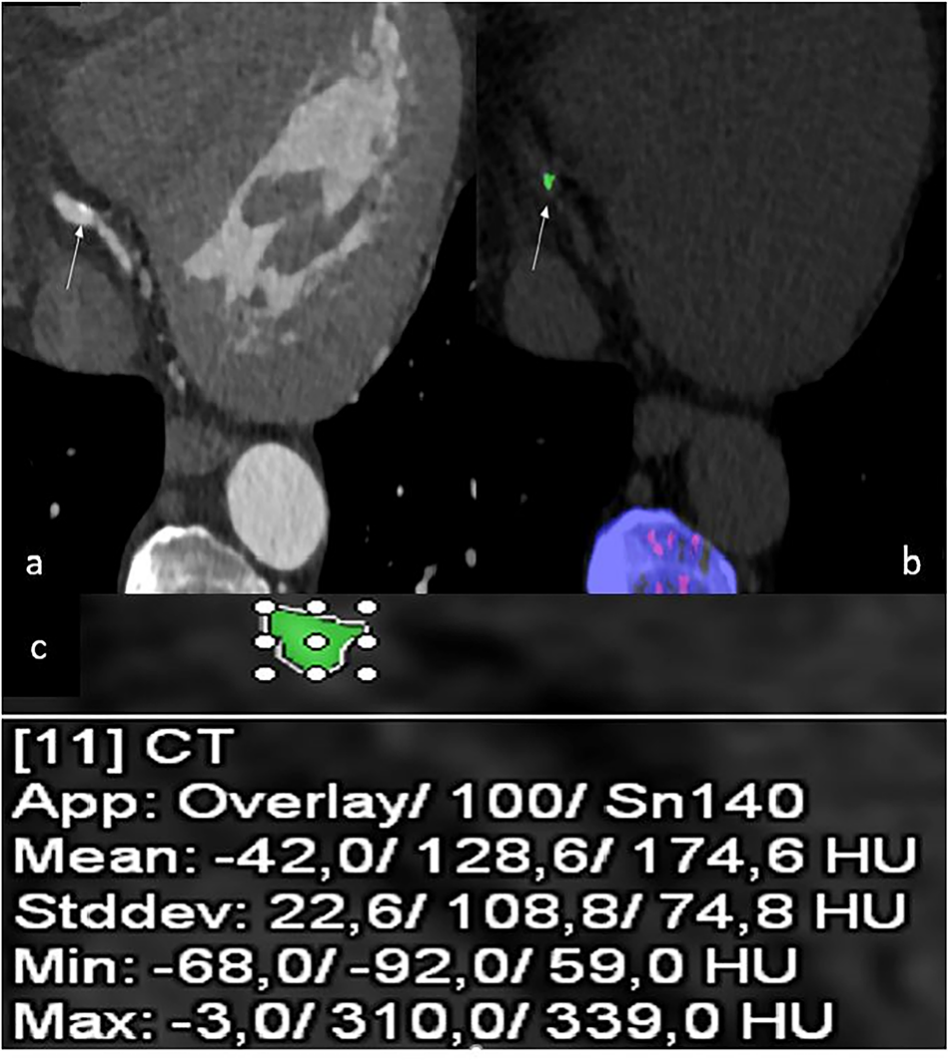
The same 63-year-old male patient with gout presented in Fig. 1, here shown for a calcified plaque in the distal RCA (arrow in a), also correlated with an MSU-positive lesion (arrow in b). Coronary CTA axial view (a) and DECT scan (b). Calculated density and volume of the MSU-positive lesions (c). Green = MSU-positive lesion. CTA, Computed tomography angiography; DECT, Dual-energy computed tomography; MSU, Monosodium urate; RCA, Right coronary artery
